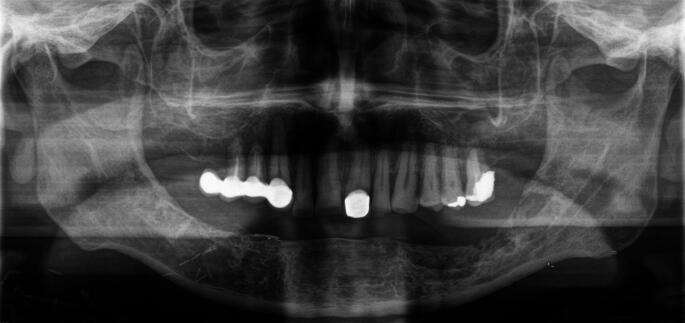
Orthopantomogram (OPG) at the initial presentation of a 53-year-old male patient with extensive osteoradionecrosis (ORN) in the mandible
